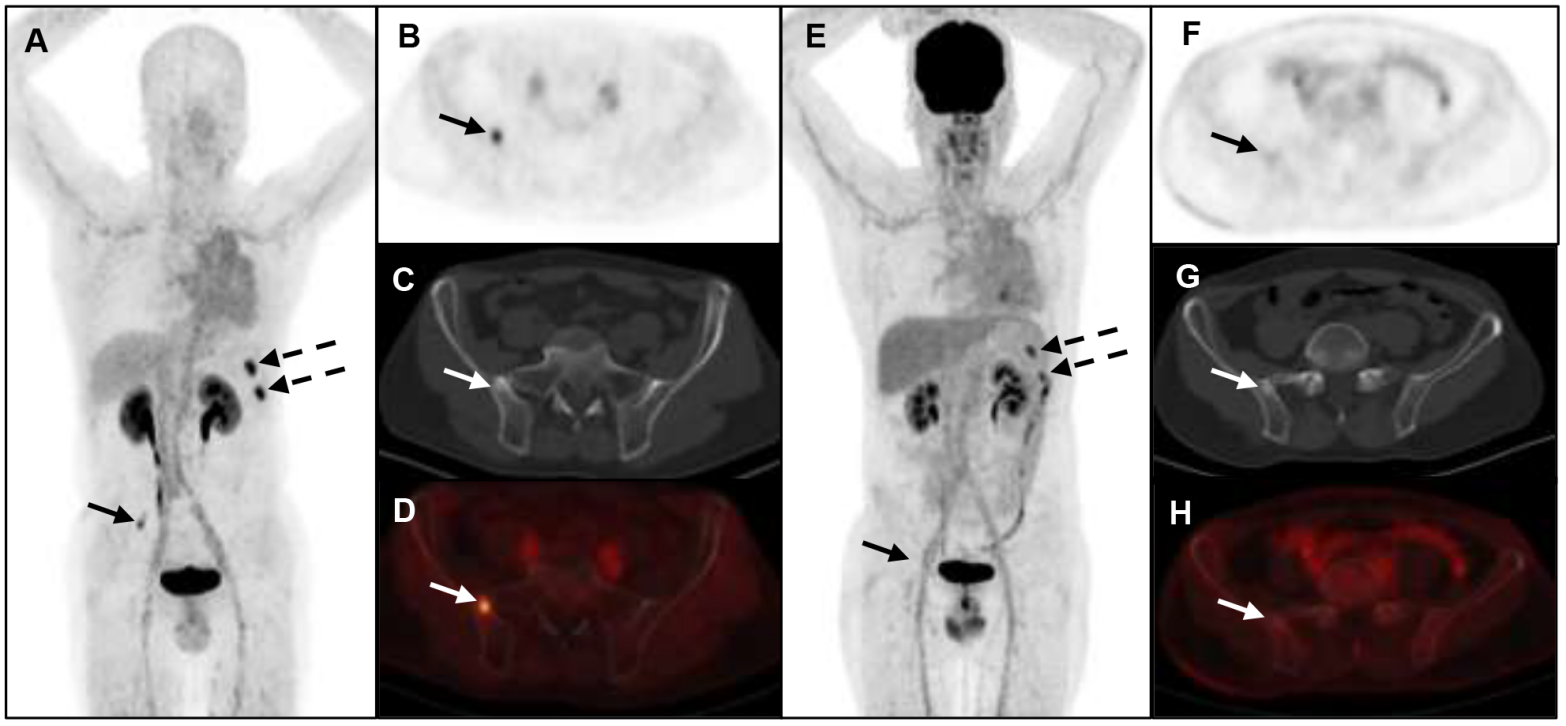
A 52-year-old man with metastatic lung cancer underwent cancer restaging imaging. ^68^Ga-FAP-2286 (A-D) uptakes in ilium (straight arrow, SUVmax 5.24) and left ribs (dotted arrow, SUVmax 8.12). ^18^F-FDG PET/CT (E-H) shows uptake in lower left ribs (dotted arrow, SUVmax 5.26), and no uptake in ilium.
